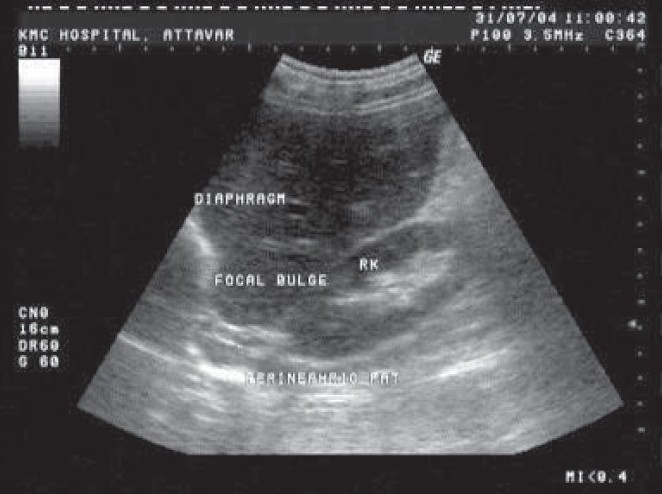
Ultrasonography showing an area of focal eventration of the diaphragm with adjacent protrusion of perinephric fat.
